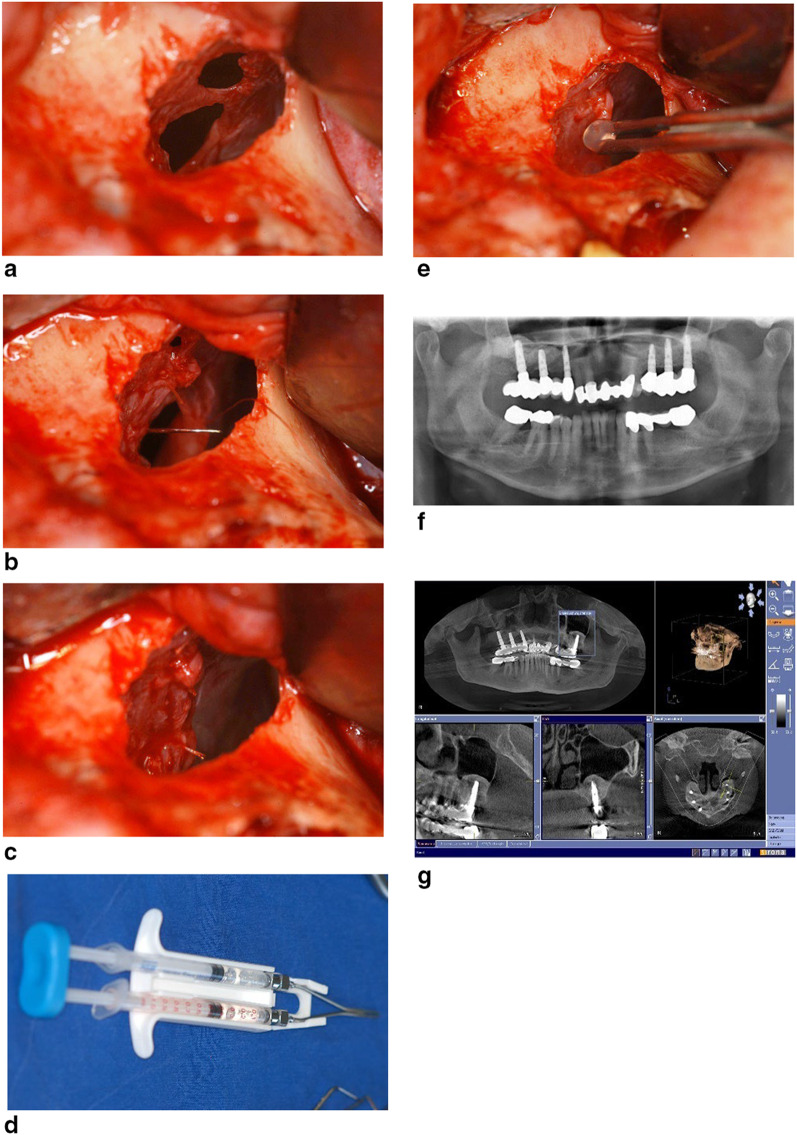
a Multiple perforation of the schneidarian membrane. b Suturing of the perforations with 70 resorbable sutures. c Hermetic closure of the perforations. d Fibrin glue syringe (Beriplast): 2 components joint together to form the adhesive. e The thin membrane is reinforced with the fibrin adhesive especially over the sutured membrane. f Control radiograph 10 years after the oral rehabilitation. g CBCT confirming the stability of the sinus graft around the osseointegrated implants with the free ostium and without any sinus pathology
